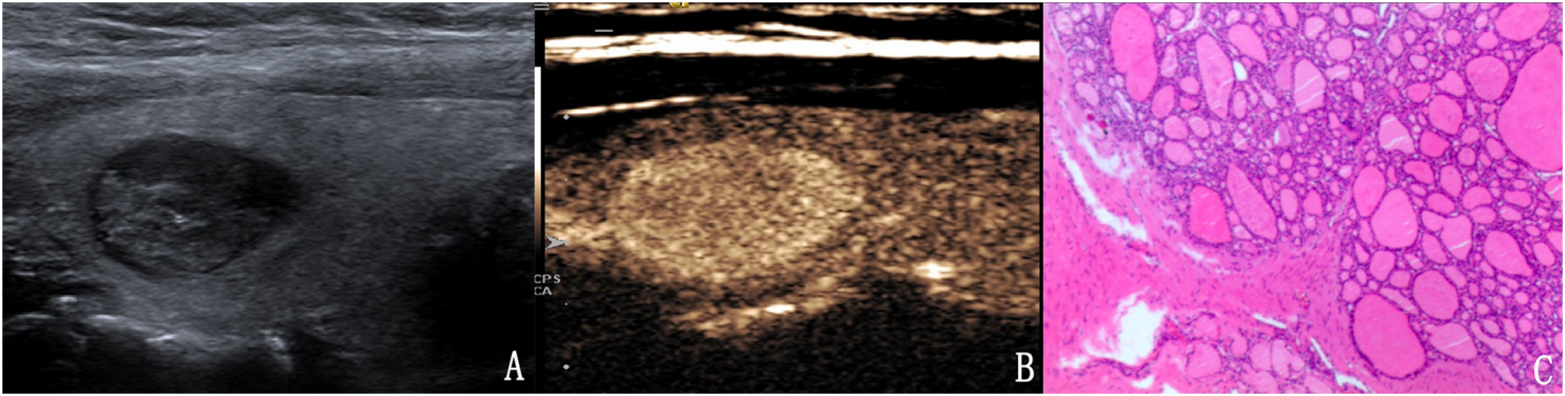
The case of a 31-year-old woman with a 15.1 × 10.1 × 9.8 mm solid hypo-echoic nodule in the right lobe of the thyroid. (A) Conventional ultrasound showed that the nodule had one malignant indicator (solid) and was classified as a TI-RADS score of 3. (B) Contrast-enhanced ultrasound detected high enhancement. The modified TI-RADS resulted in a score of 2 and indicated a benign nodule. (C) Pathology of the lesion showed an adenoma.
